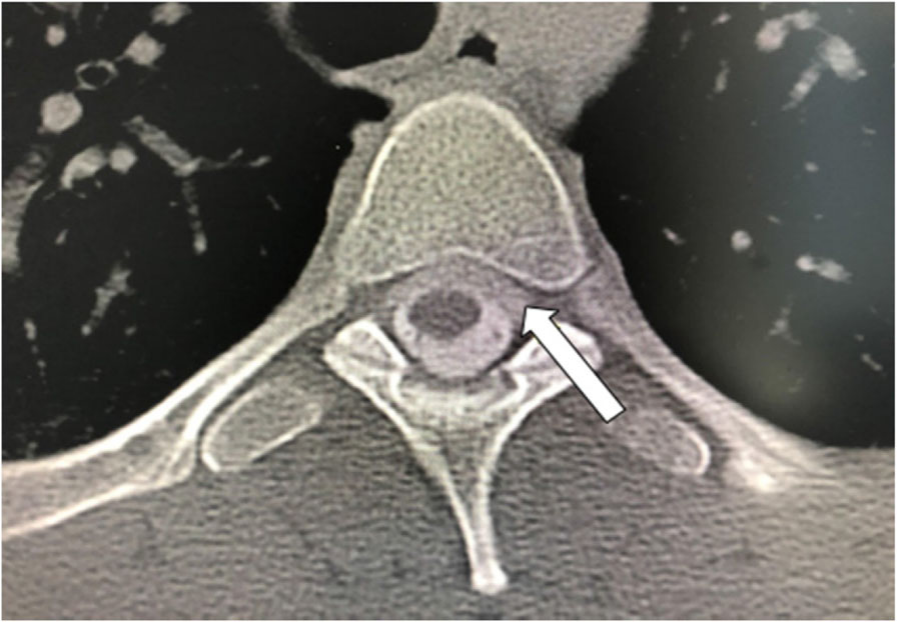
Computed Tomography Myelogram (axial view) demonstrated a ventral cervicothoracic epidural CSF collection (arrow) extending from the mid cervical spine to mid thoracic level (Patient 1)
